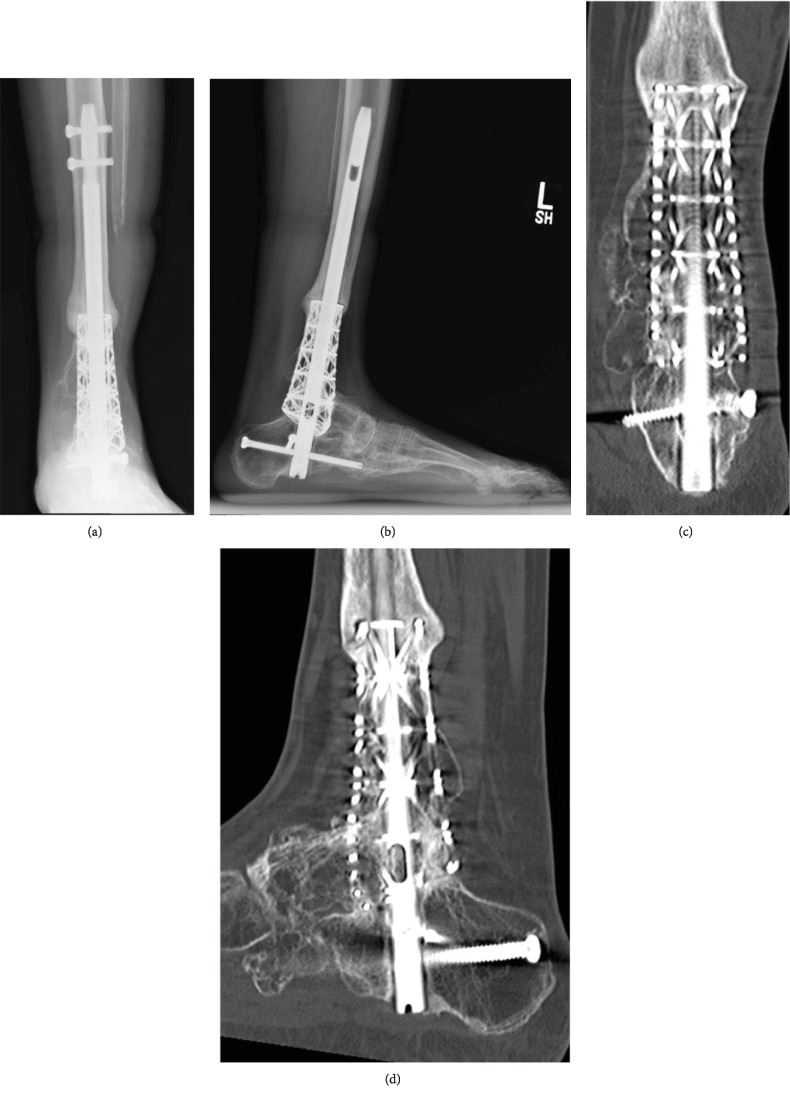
Anteroposterior (a) and lateral (b) radiographs of the lower leg and hindfoot 48 months after definitive surgery. Coronal (c) and sagittal (d) CT scan images from the same timepoint demonstrating bone growth into the cage from the tibia, talus, and calcaneus.
